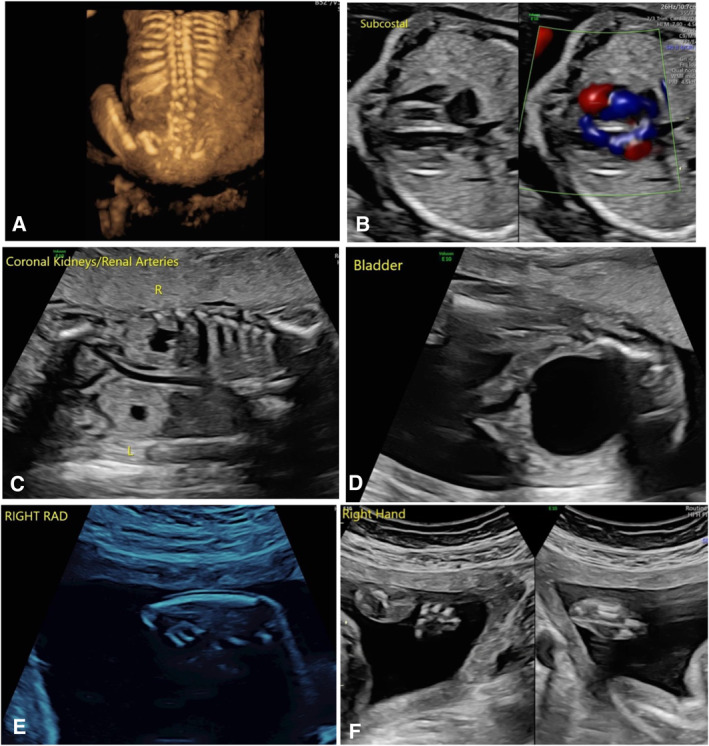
Prenatal ultrasound findings at 20 weeks' gestation. (A) Lumbar hemivertebra. (B) Ventricular septal defect, subcostal view. (C) Dilated renal pelvis bilaterally, left renal pelvis 8.7 mm, right 6.1 mm (measurement not shown). (D) Enlarged fetal bladder and single umbilical artery. (E) Single right forearm bone and radial curvature of the right hand. (F) Right hand.
